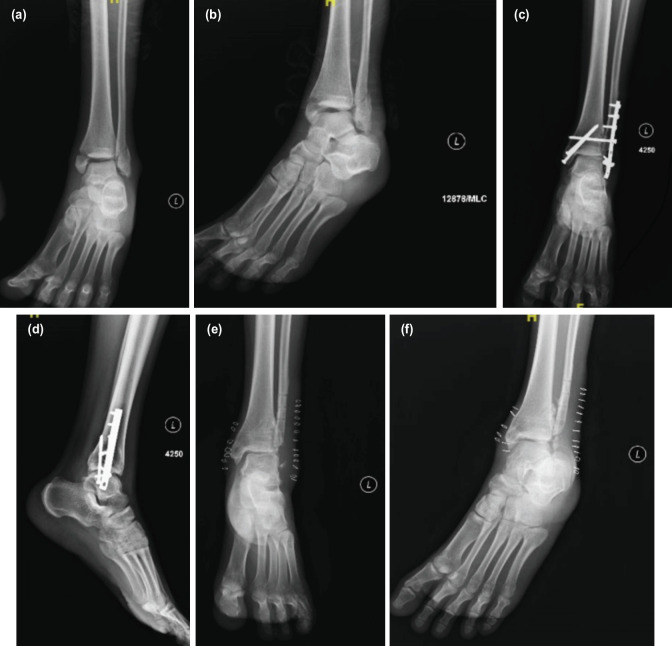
(a, b) Pre-operative radiograph of a 22-year-old male with closed bimalleolar ankle fracture who was operated. (c, d) Shows immediate post-operative radiographs. (e, f) Shows destruction of distal end of lateral malleolus secondary to infection for which implant removal was done.
